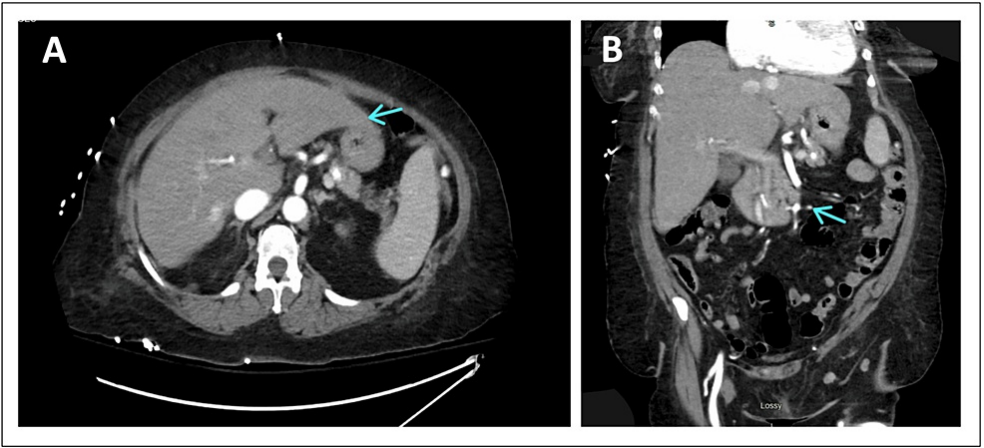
CT of the Abdomen and Pelvis (A) Axial view. (B) Coronal view. No acute findings in the abdomen or pelvis were found. Both arrows indicate areas of normal abdominal findings with no acute changes. CT: computed tomography
